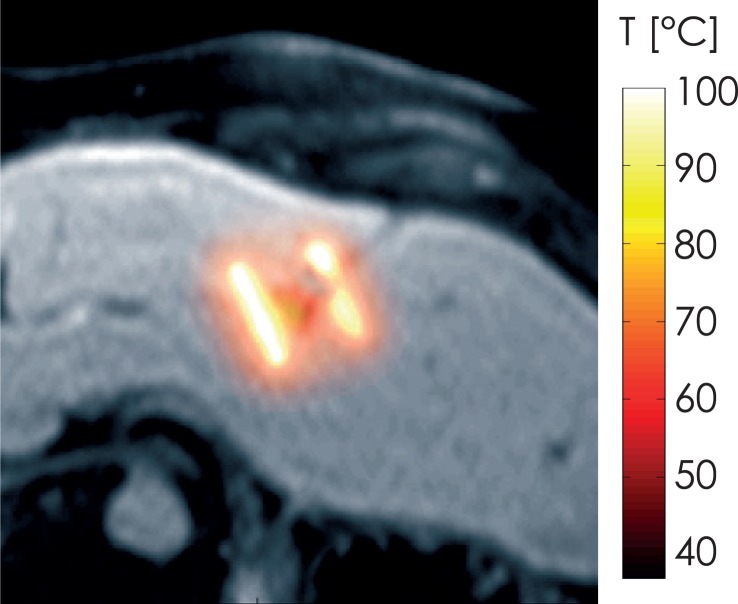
One slice showing computed temperature distribution after all pulses from the 7^st^ pulse train (electrode pair 1 – 2 at 3000 V) superimposed on the corresponding MRI slice of the model.
